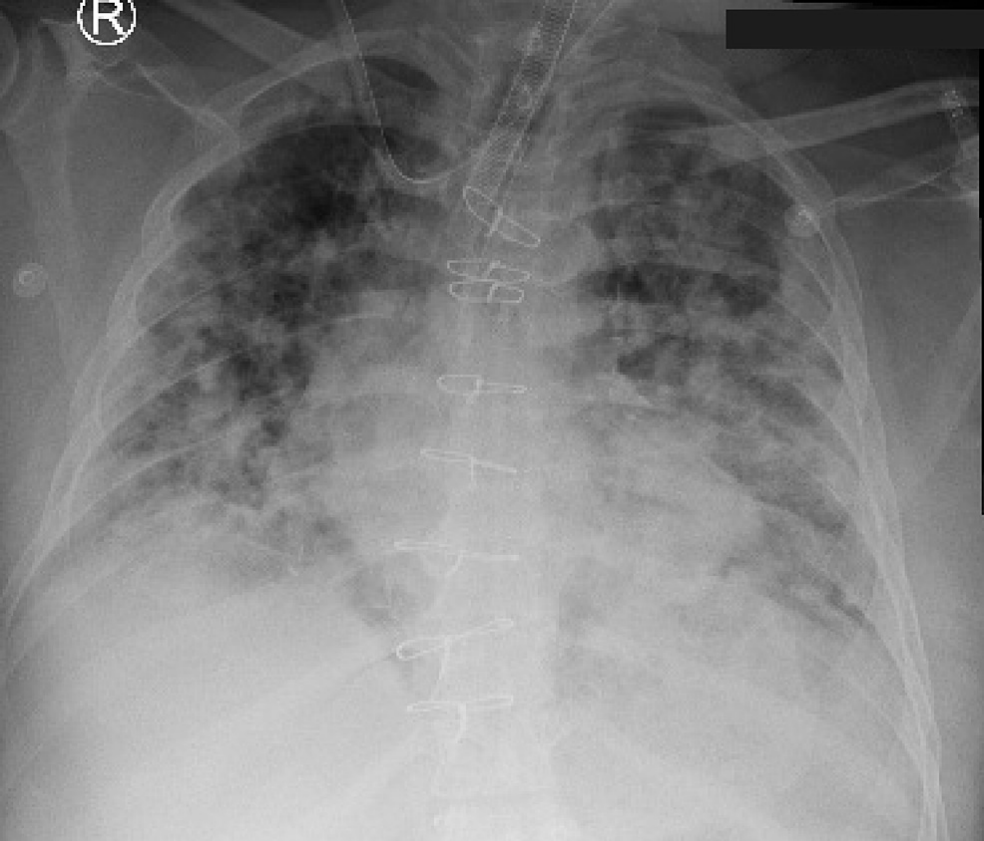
Chest x-ray of the patient during admission
